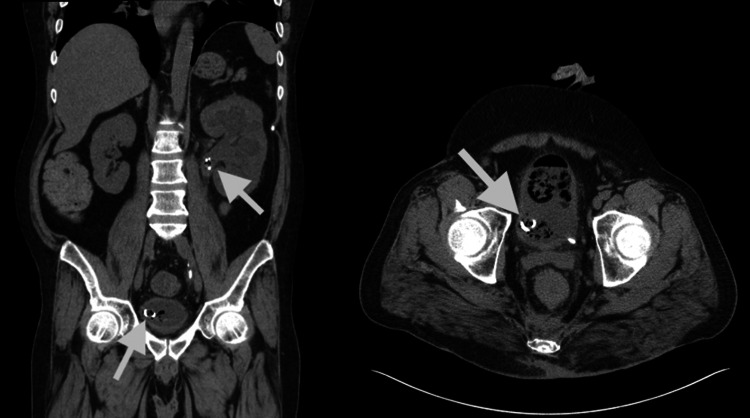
Abdominal CT scan showing left hydronephrosis with left ureteral stent in place extending from the left renal pelvis to the urinary bladder. Heterogenous filling defects containing gas noted in the urinary bladder (arrows).
